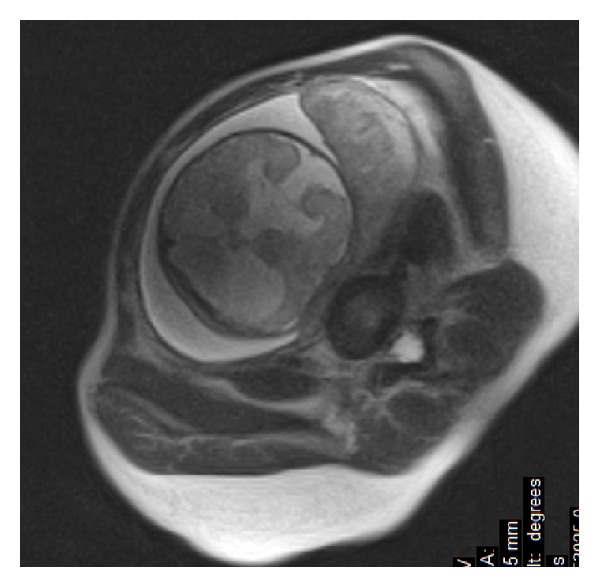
Fetal MRI. SSFSE, T2WI, coronal plane. Agenesis of the septum pellucidum and of the corpus callosum.
